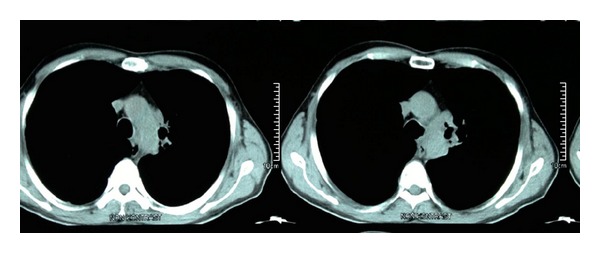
A mass lesion constricting the upper left lobe bronchus with hazy boundaries with the descending aorta observed in the thoracic CT.
